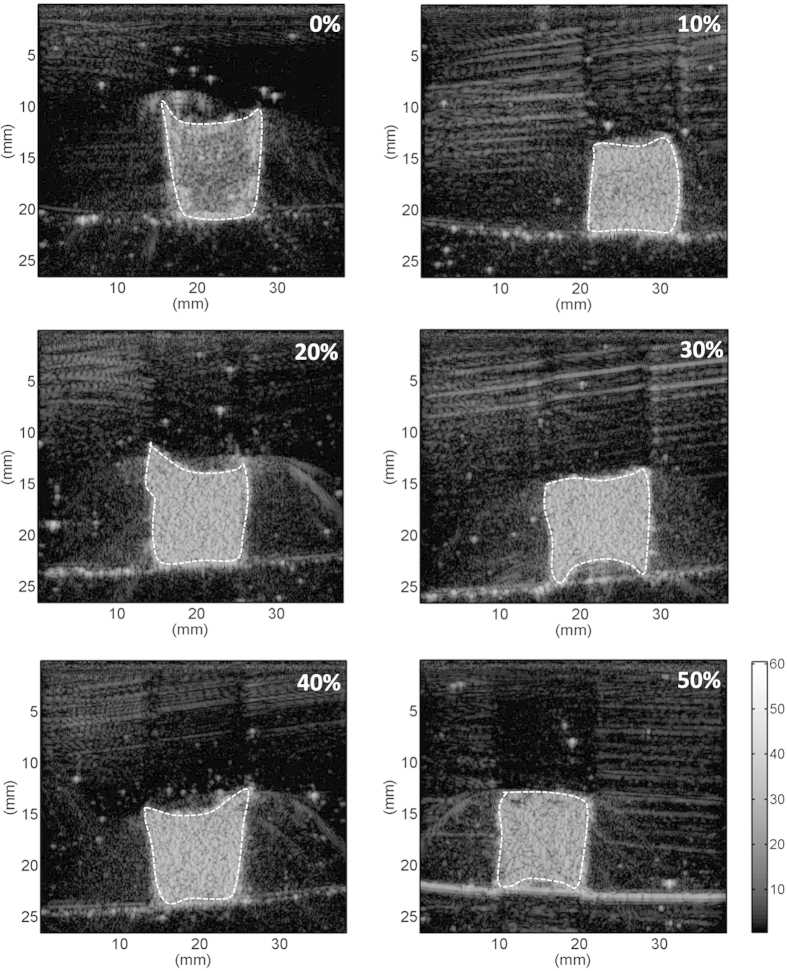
Ultrasonic B-mode images of thrombi with different hematocrits. The brightness of the B-mode images increased as the hematocrit increased from 0% to a maximum of 20%. Beyond the hematocrit of 20%, the brightness of the B-mode images decreased slightly. Whole blood samples were liquid; hence, no images are available.
